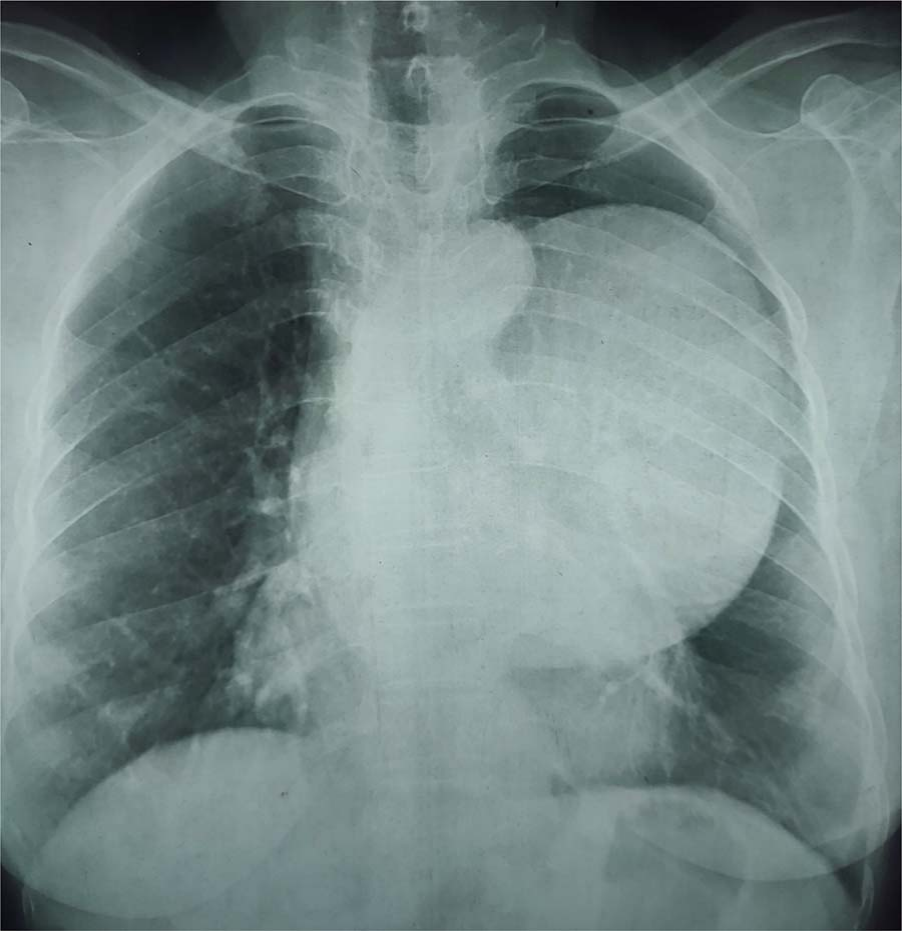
Chest X-ray (PA view) showing large, well-defined homogenous opacity in the left middle lung zone, causing silhouetting of the left hemidiaphragm and cardiac border.
